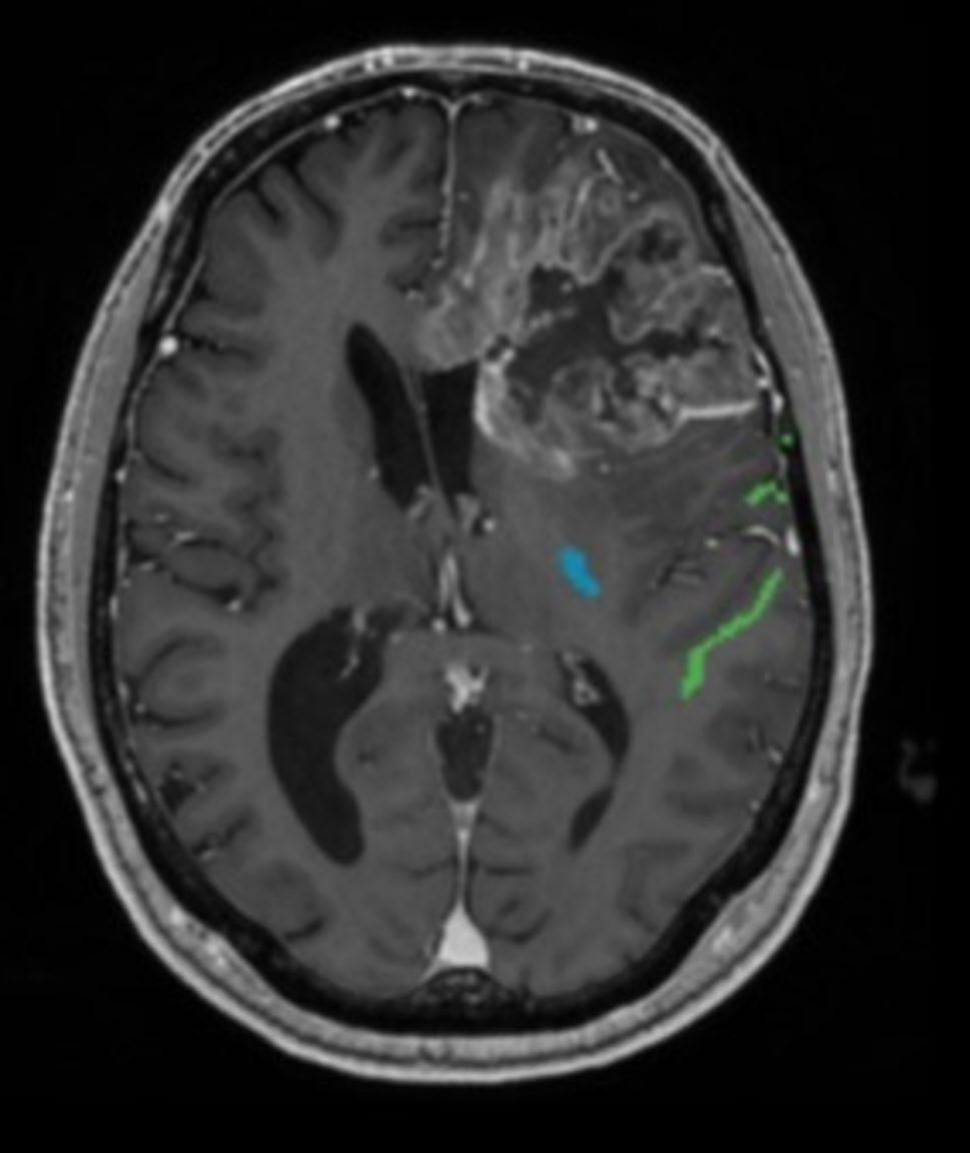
MRI showing a contrast enhancing tumour in the left frontal lobe. Corticospinal tract = blue, arcuate fascicle = green
